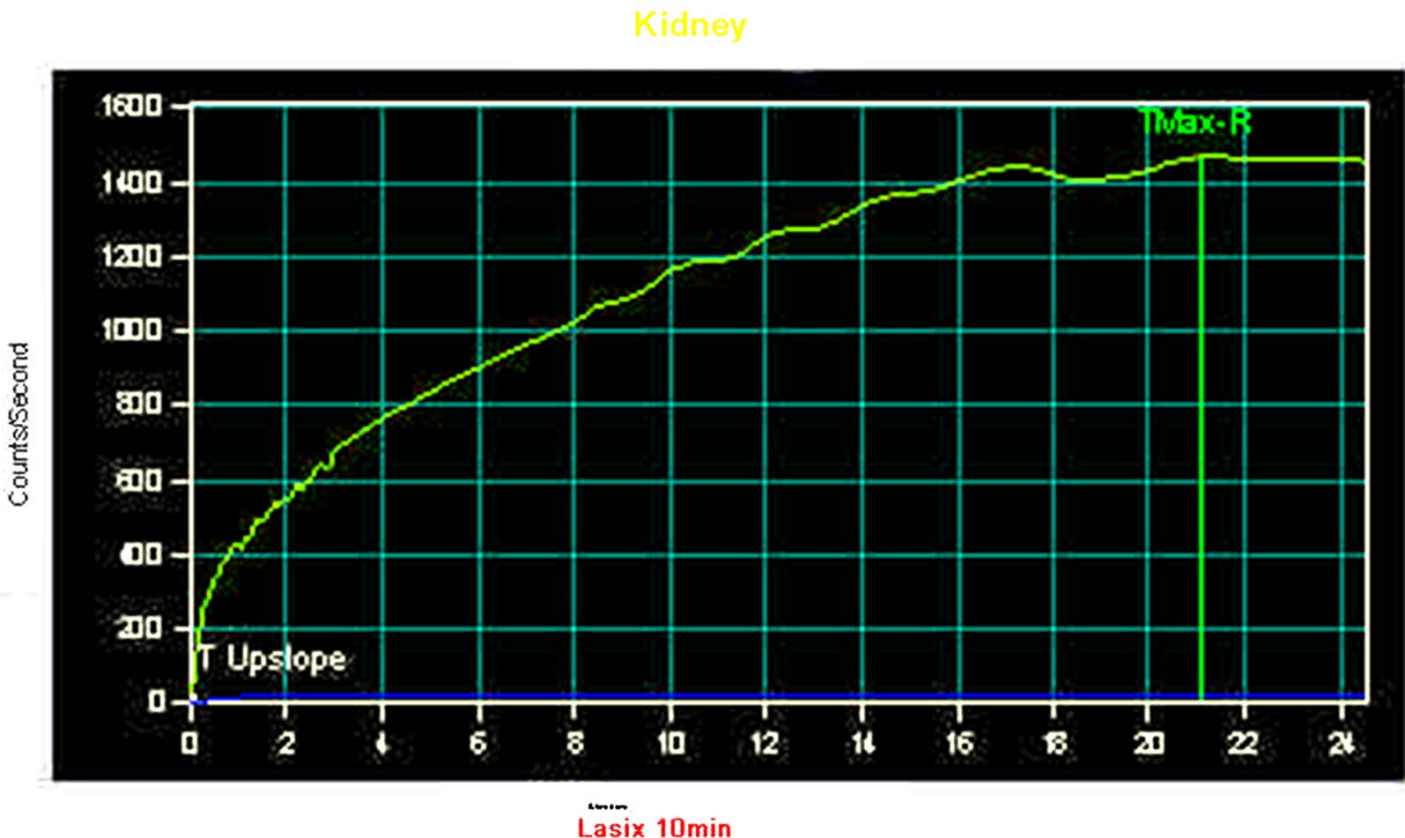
Preoperative renal scintigraphy findings
